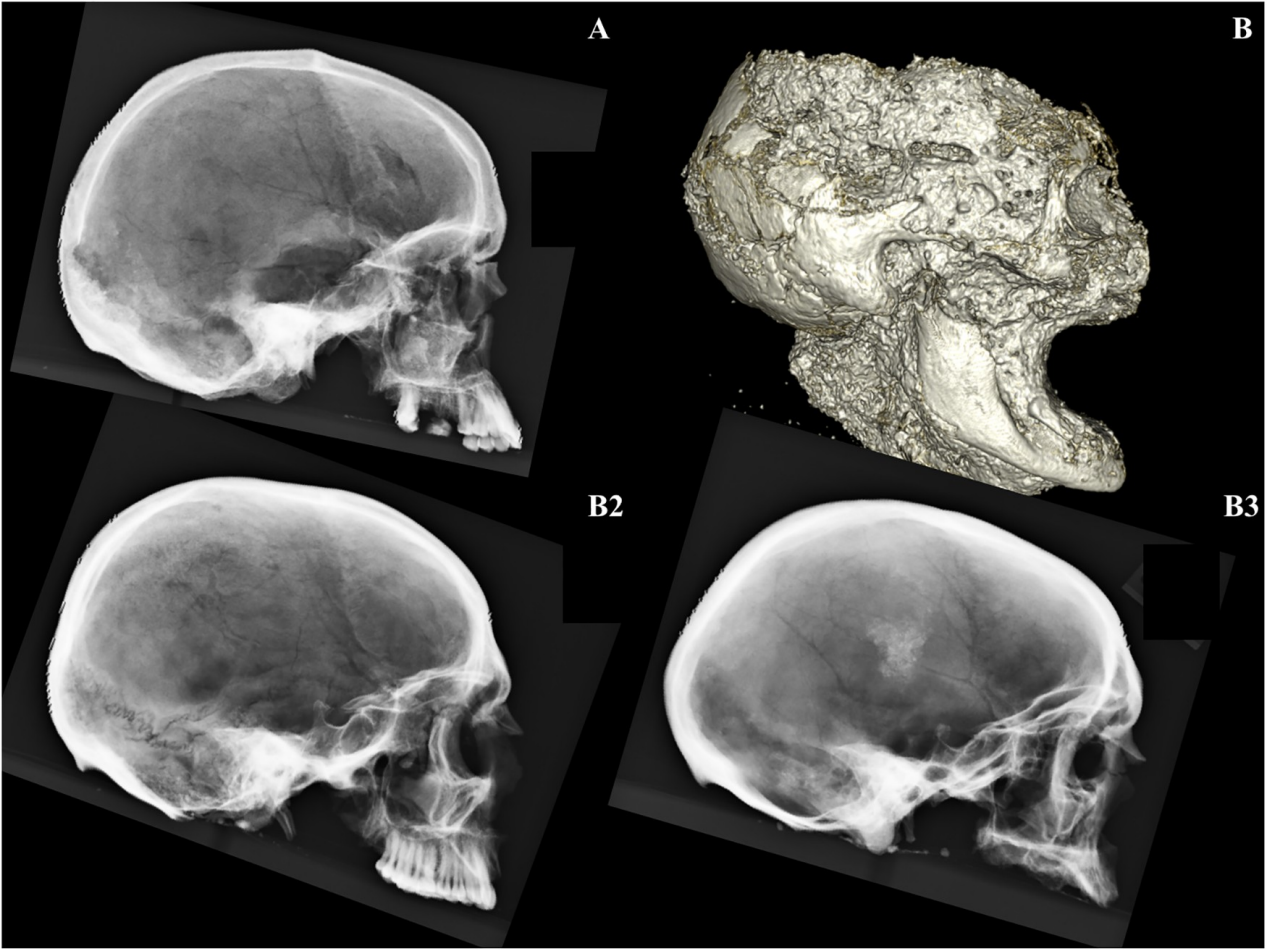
YNH4 lateral radiographs. YNH4 A has a damaged splanchnocranium. The fragile condition of the YNH4 B skull required a CT scan while still in matrix. Radiographs by GJC, TG and NAP
